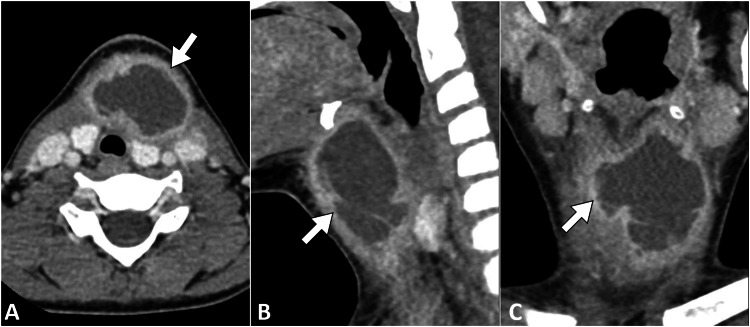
Enhanced CT images of the neck in axial (A), sagittal (B), and coronal (C) planes reveal a distinct collection (arrow) with an enhanced wall close to the thyroid gland. These findings are indicative of a fourth-type branchial cleft cyst. CT: computed tomography
